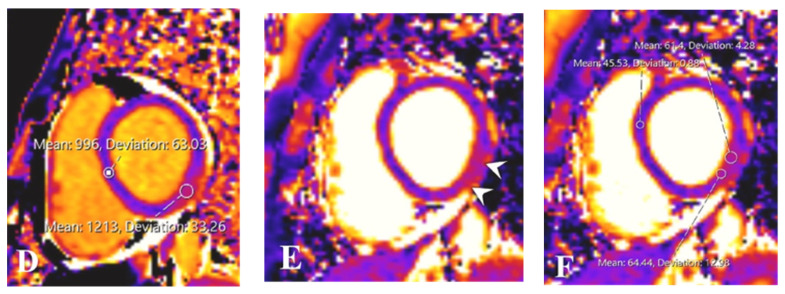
Magnetic Resonance Imaging of Case 2. Post-contrast magnitude inversion recovery (MAG-IR) (A) and phase-sensitive inversion recovery (PSIR) (B) images in short-axis views show subepicardial enhancement in the inferolateral wall at the base (arrowheads). Native T1 map shows corresponding abnormality (arrowheads in (C)) with elevated values (D) in the inferolateral wall compared with the interventricular septum. T2 mapping also showed abnormality in this region (arrows in (E)) with elevated values (F) when compared with the interventricular septum. Reprinted/adapted with permission from Ref. [15] 2021, Elsevier.
